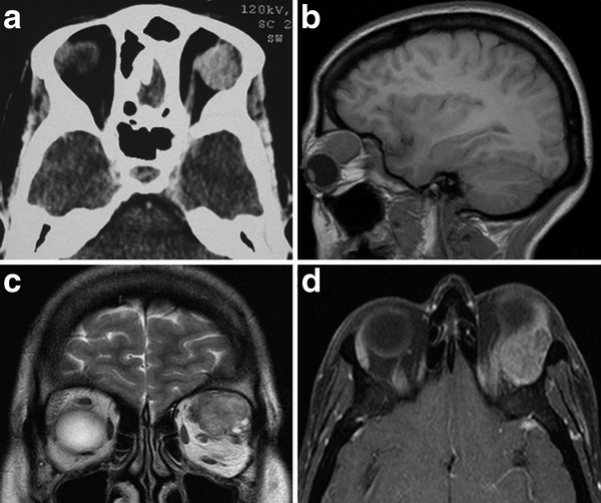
(A): Cranial CT scan demontrated an intra-orbital tumor, well circumscribed with homogeneous enhancement without bone erosion. (B, C and D): MRI showed a round mass that was extraconal, isointensity on T1-weighted imaging, slightly hyperintensity on T2-weighted imaging and enhanced after intravenous contrast administration.
